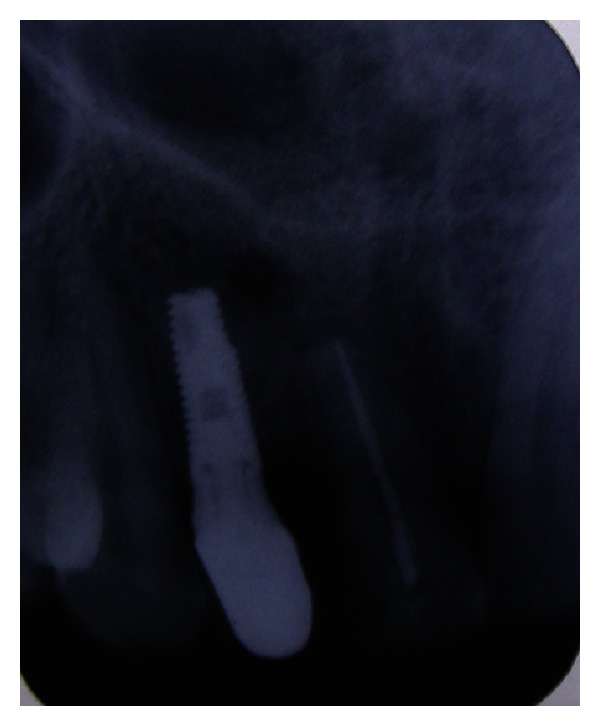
IOPA radiograph of the restored site 1 year after loading.
